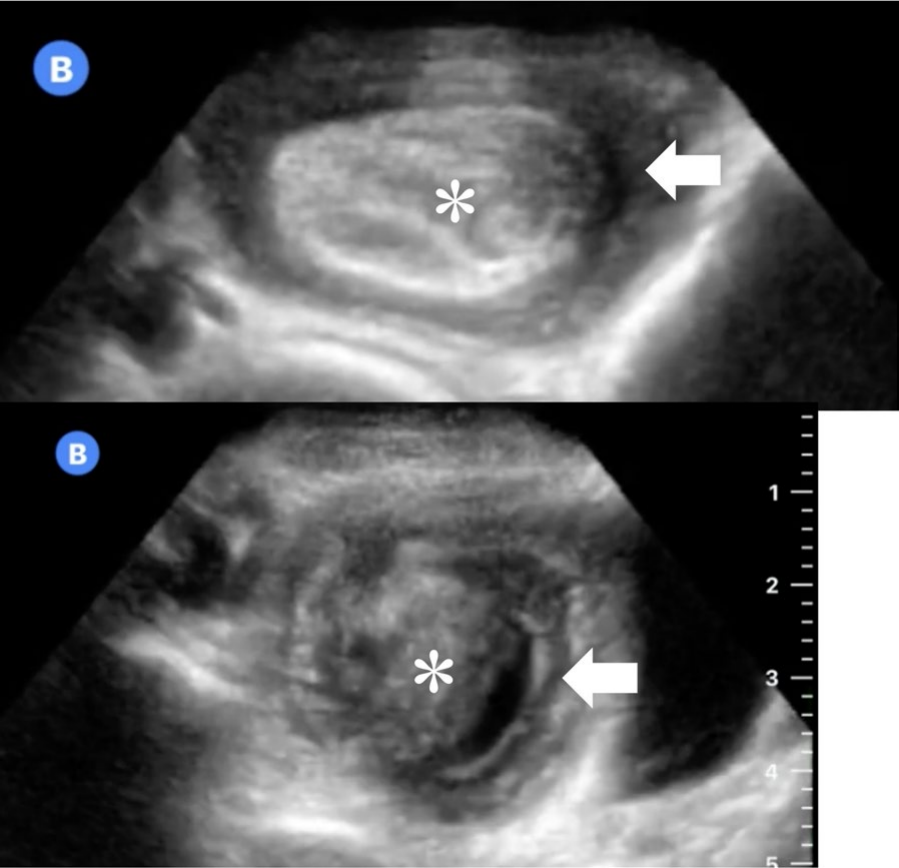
Abdominal point-of-care ultrasound of a previously healthy 3-year-old male with abdominal pain, nausea, and vomiting diagnosed with intussusception, confirmed and treated by air contrast enema. a reveals a stereotypical “target sign”, hyperechoic compressed inner loop of bowel (*) telescoping within a hypoechoic, edematous outer loop (arrow). b reveals the target sign in another cross sectional plane, with multiple layers of telescoping bowel
